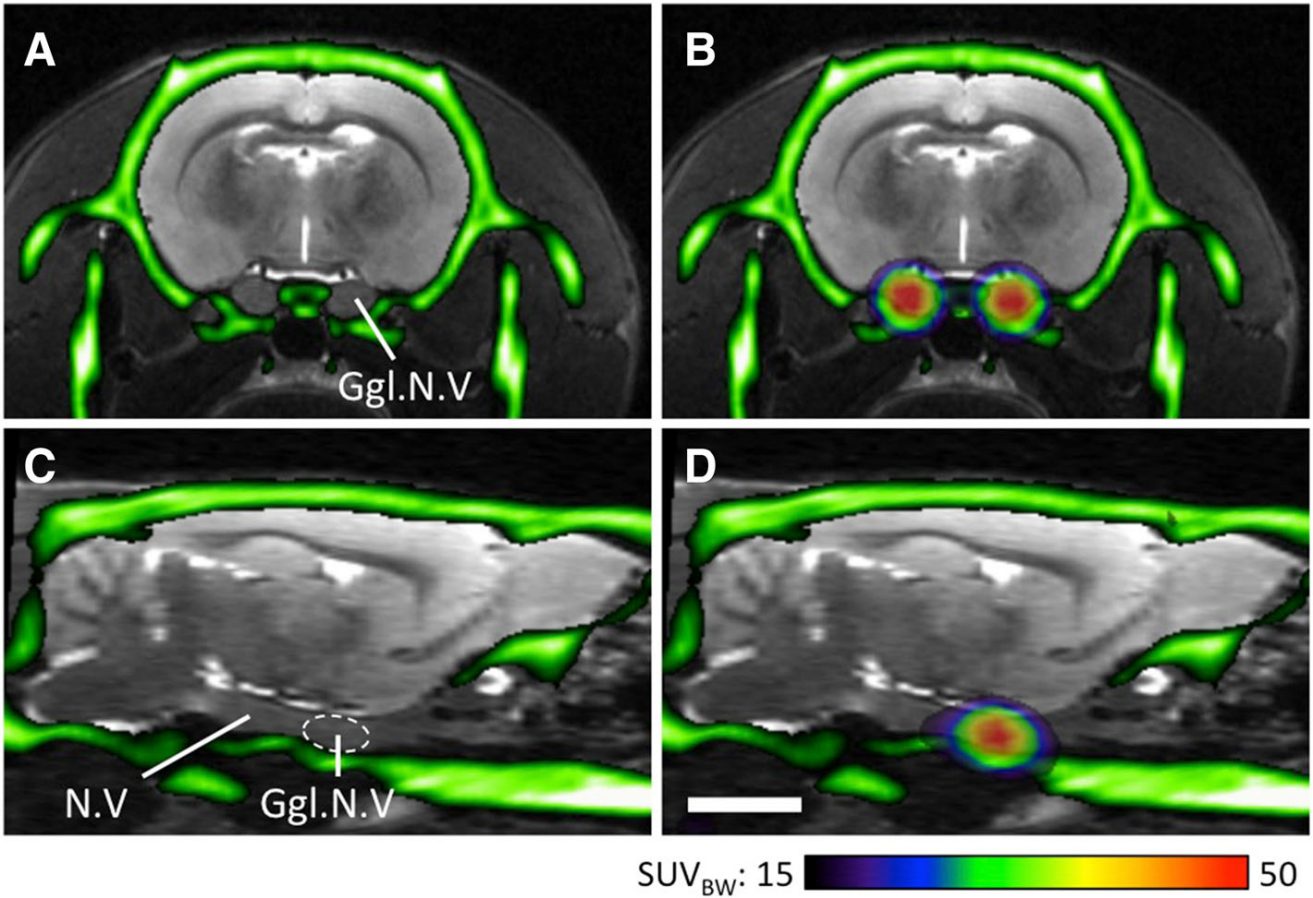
[^18^F]DCFPyl accumulation in the trigeminal ganglion. (a) + (c): Overlay of the CT image (green) and an MRI template (grey) in the transverse (a) and sagittal plane (c). (b) + (d): Overlay between CT (green), MRI (grey) and PET (colored). Abbreviations: Ggl.N.V: trigeminal ganglion; N.V: trigeminal nerve. Scale bar: 5 mm
